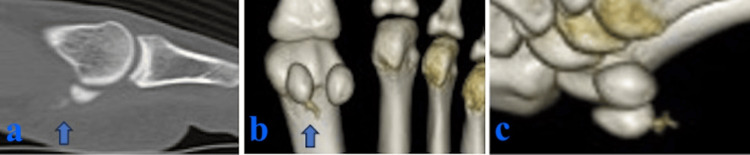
CT scans (a) Sagittal view. (b, c) 3D CT images. The arrows indicate calcification in the flexor hallucis brevis.
